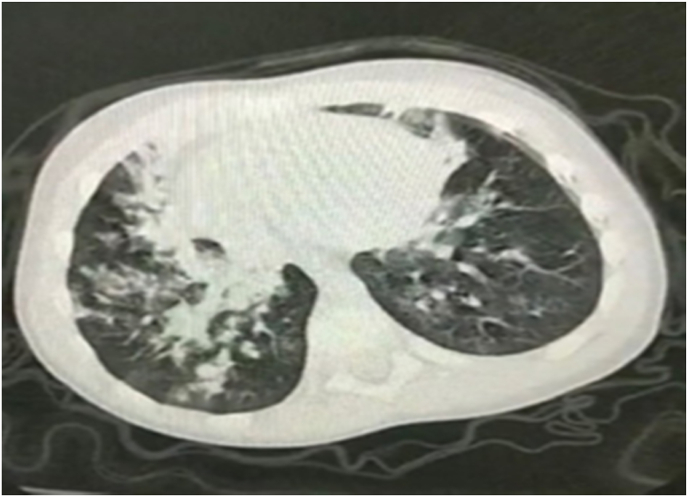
Thoracic CT scan showing lung damage of 30%.
